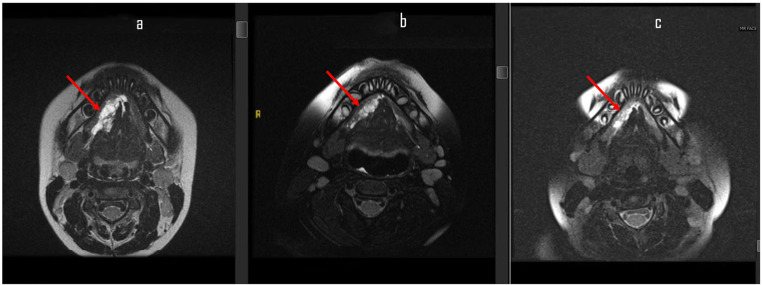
a, b, c: MRI Axial T2 at Diagnosis, after Six Months into Therapy, and 6 Months Post Completion of Therapy
